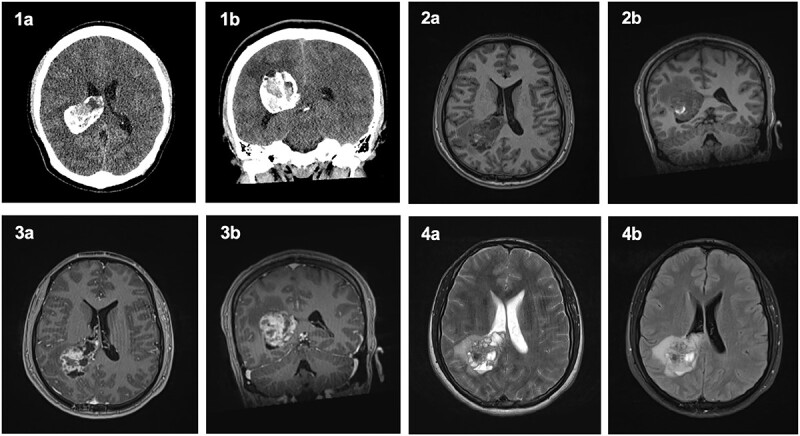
Computed Tomography and Magnetic Resonance Imaging showing a mass within the trigone of the right lateral ventricle. On CT, axial (1a) and coronal (1b) sections revealed a calcified mass with a central cystic component. Pre-contrast T1-weighted imaging showed an isointense mass with a central ring of enhancement on axial (2a) and coronal (2b) slices. Post-contrast T1-weighted imaging with irregular enhancement of the lesion on axial (3a) and coronal (3b) slices. Axial T2-weighted imaging (4a) and FLAIR (4b) exhibited an isointense lesion with surrounding oedema.
